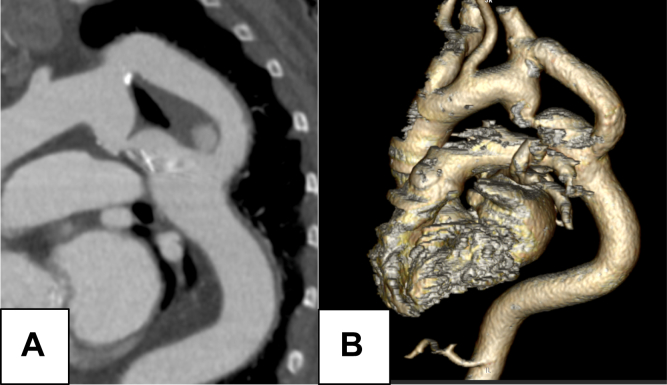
Preoperative CT angiography. Contrast-enhanced CT angiography showing a pseudoaneurysm arising from the distal anastomosis of the extra-anatomic graft. The native coarctation site remains patent and may contribute to retrograde flow into the pseudoaneurysm sac (A). CT angiography reconstruction of aortic coarctation (B). *CT*, Computed tomography.
